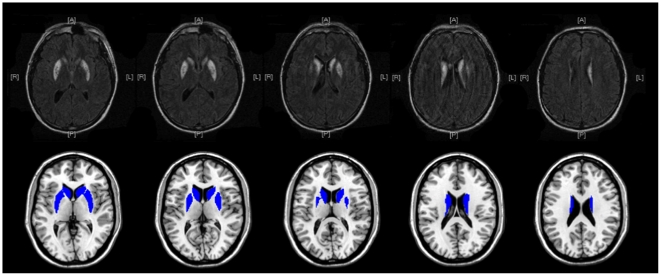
FLAIR MRI images from XG demonstrating injury to the caudate, putamen and globus pallidus; white regions indicate areas of damage. Overlay images of the intact basal ganglia on a template brain are displayed for reference purposes.
